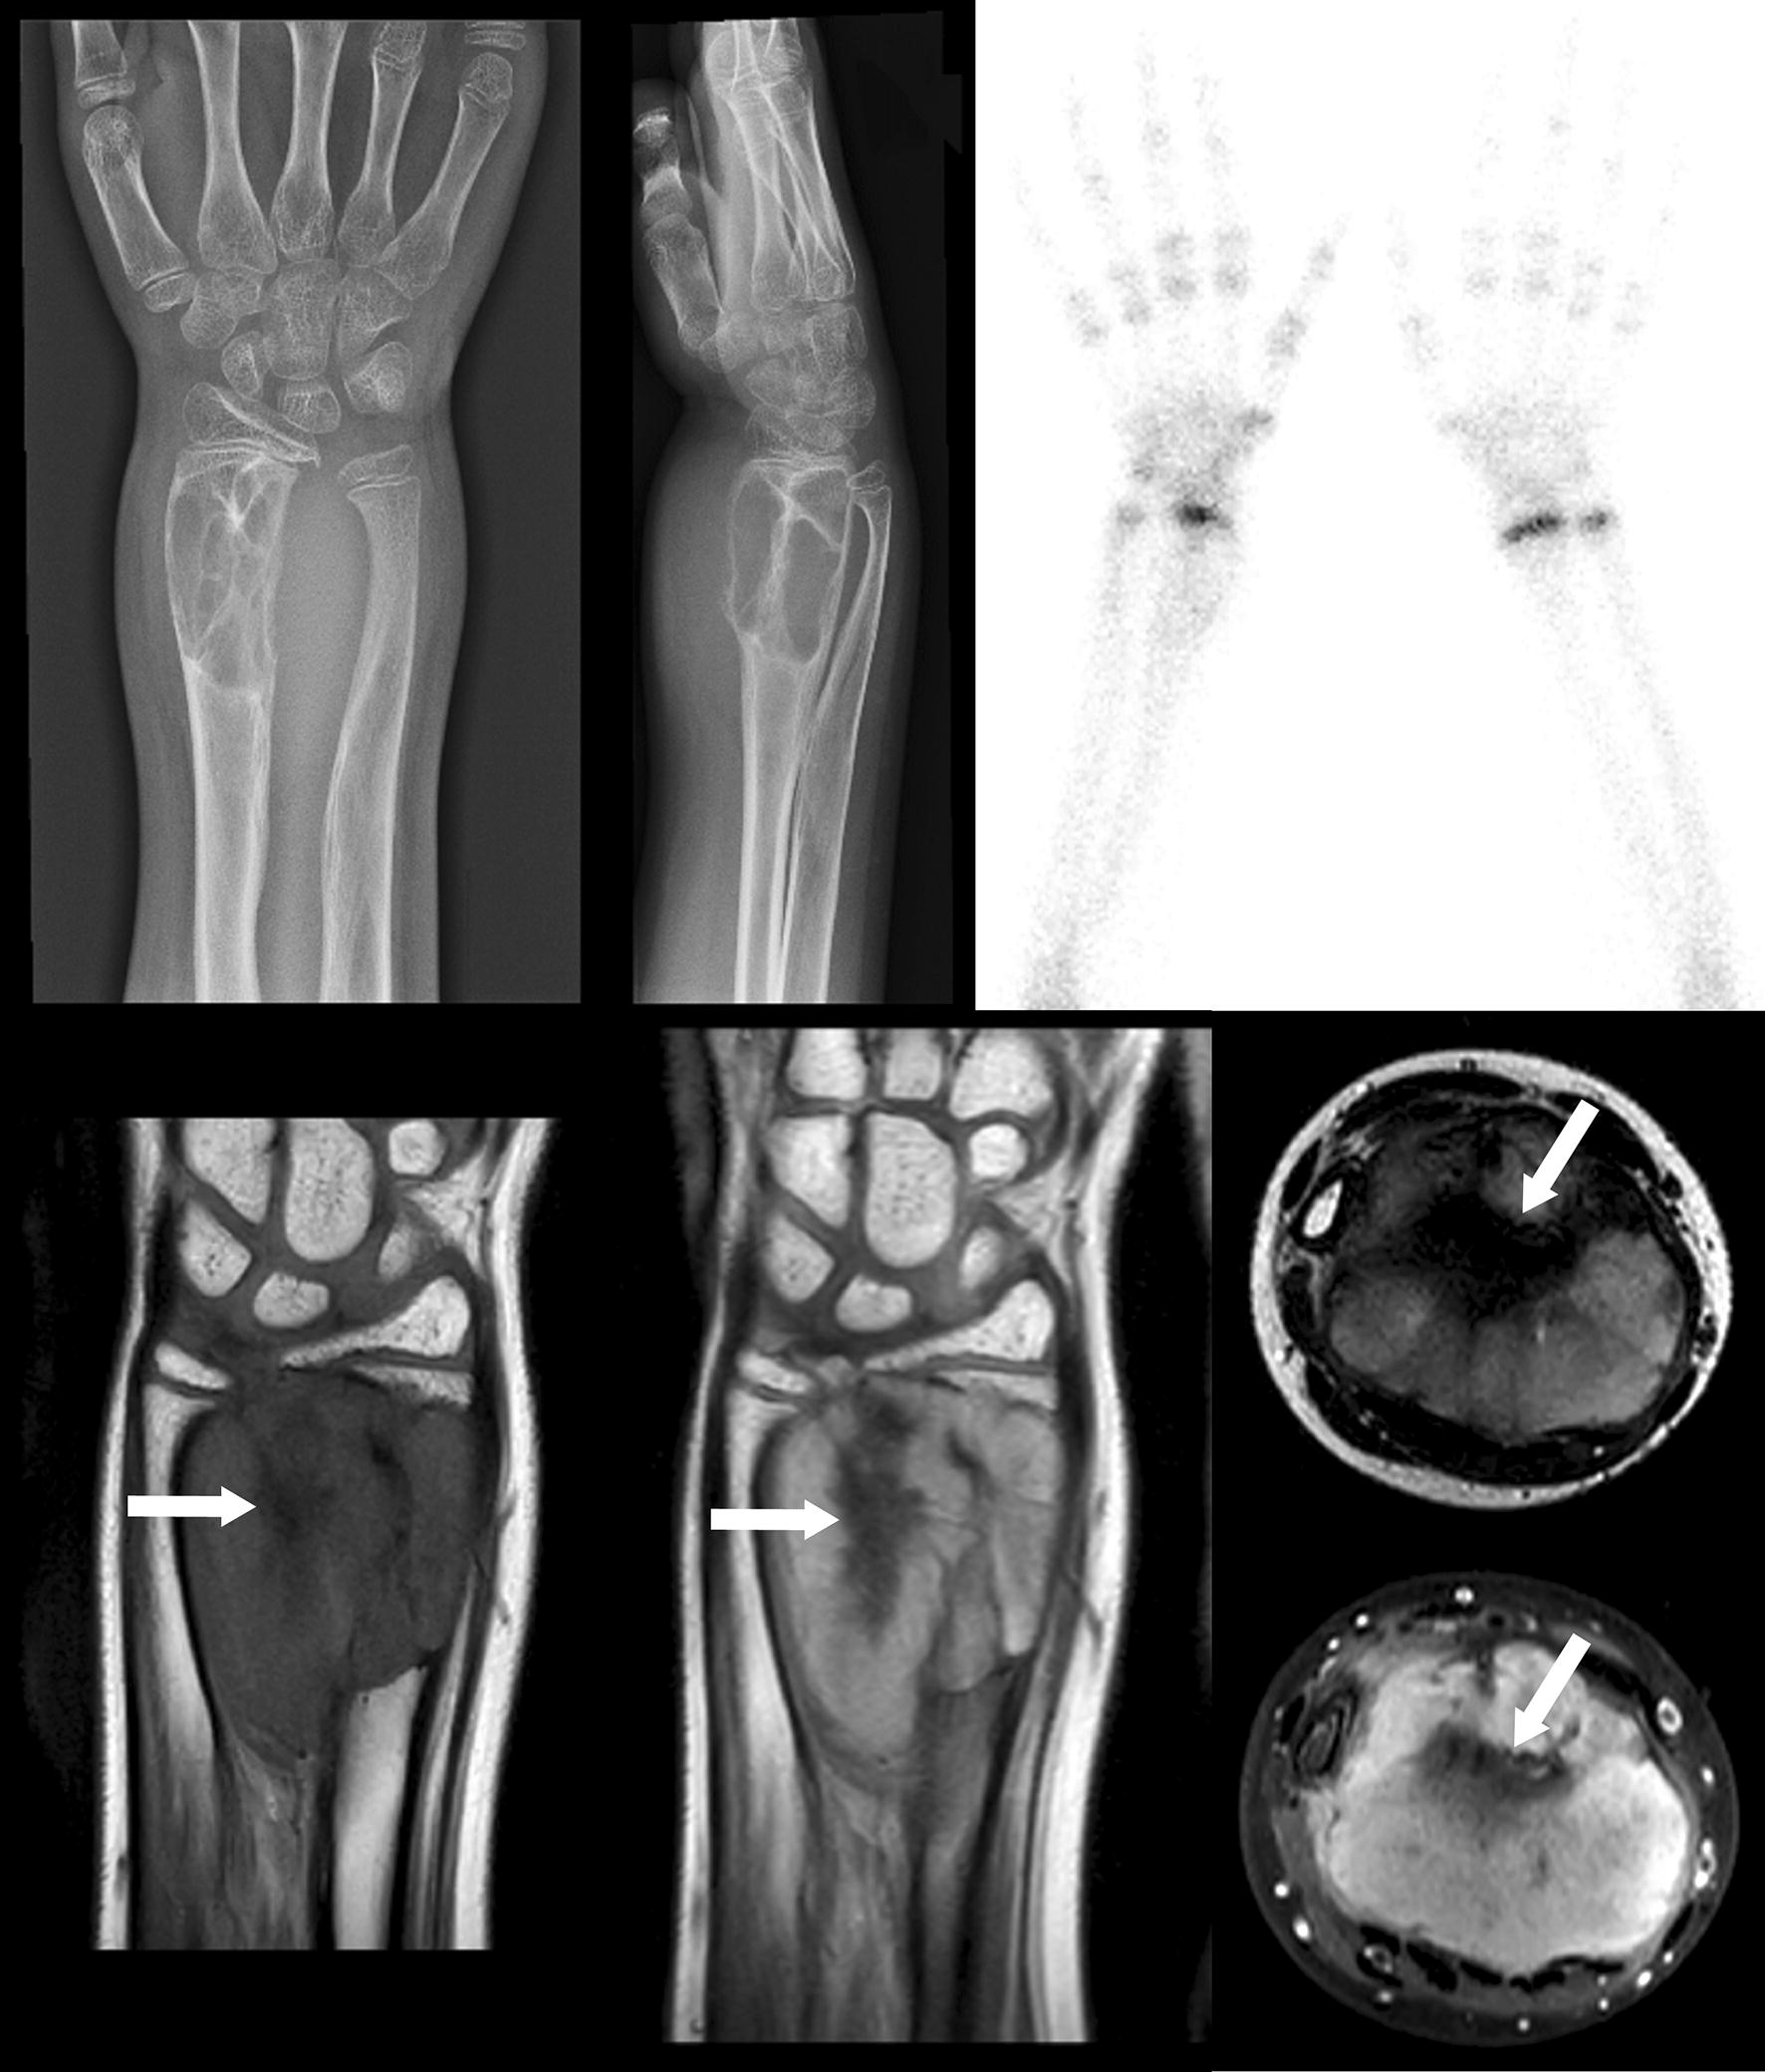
Conventional AP and lateral radiographs (top left and top middle) show an expansive bubbly lytic bone lesion in the diaphysis-metaphysis of the right distal radius with a narrow zone of transition, nonsclerotic margins, cortical thinning and destruction, and an accompanying large soft-tissue mass which appears to compress the distal ulna with bowing of the latter. Bone scintigraphy (top right) shows no increased uptake at the location of the lesion. MRI with coronal T1-weighted (bottom left) and gadolinium-enhanced T1-weighted (bottom middle) images, and axial T2-weighted and gadolinium-enhanced fat-suppressed T1-weighted images (bottom right) are in keeping with the conventional radiographic findings, and also demonstrate no signs of invasion in surrounding muscles or ulna. Remarkably, in the center of the lesion there is low signal on all sequences (arrows), most strikingly on the T2-weighted sequence. Because the combined imaging features suggest a slow-growing (most likely benign) process with fibrotic components, the differential diagnostic considerations include desmoplastic fibroma, and (less likely) giant-cell tumor or fibrous dysplasia
